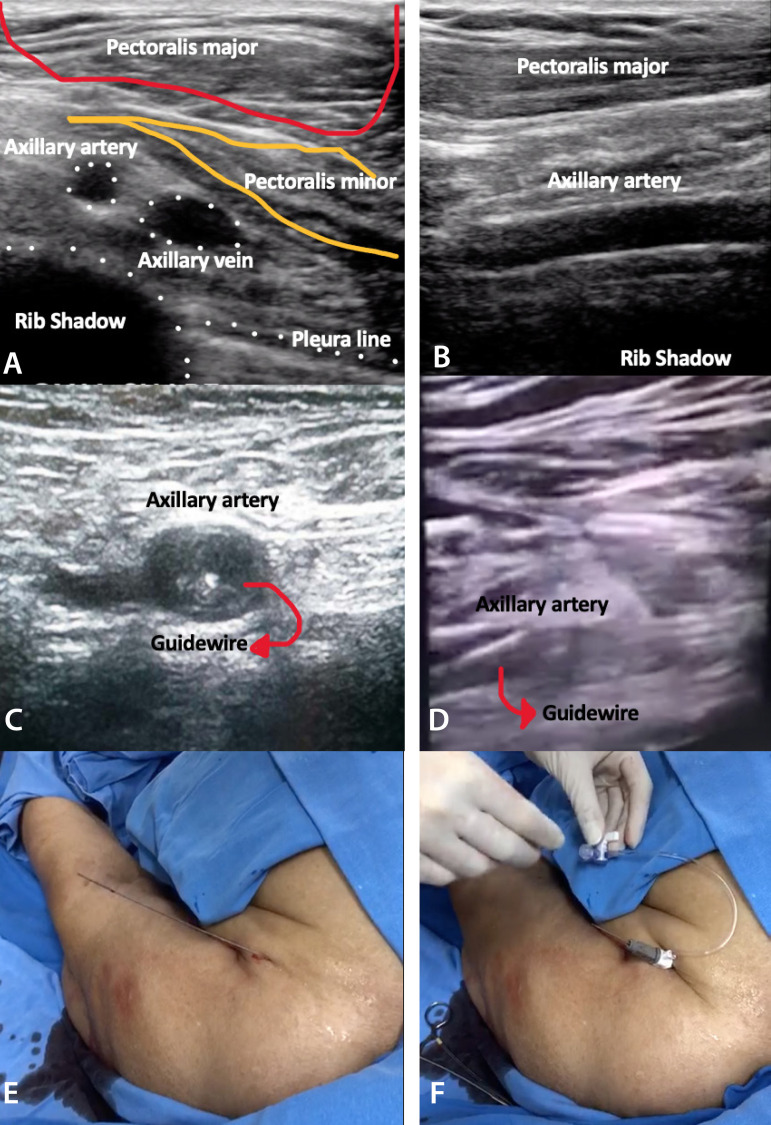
Ultrasound-guided axillary artery puncture. Ultrasound short (A) and long-axis (B) axillary artery evaluation. Out-of-plane (shot-axis) (C) and in-plane (longitudinal-axis) axillary artery puncture (D). Guidewire (E) and short sheath insertion (F).
